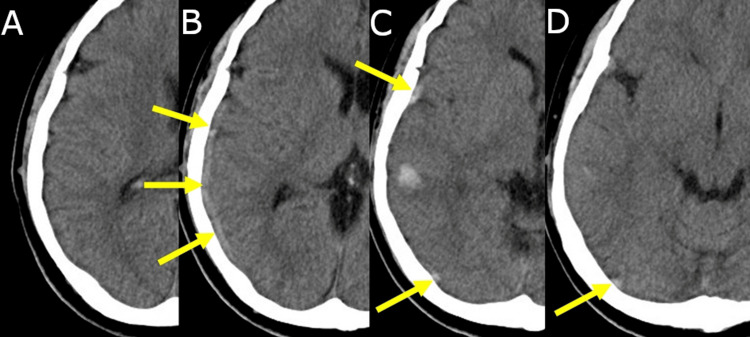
Noncontrast CT suggestive of ASDH along the right temporal convexity Initial noncontrast CT images (A-D) reveal a linear hyperdense area along the lateral convexity of the right temporal lobe (yellow arrows), which was initially interpreted as an ASDH with cortical hemorrhage. The hyperdensity is most prominent in slices B and C and remains visible through slice D. ASDH, acute subdural hematoma
